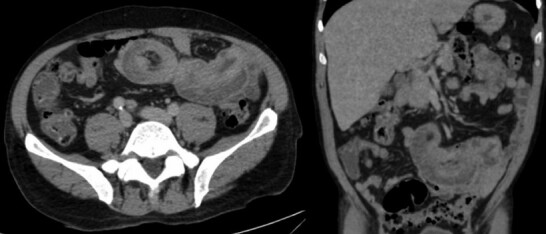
Computed tomography scan of the abdomen showing intestinal intussusception.
